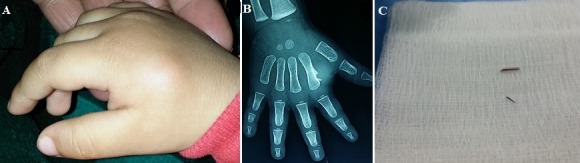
(A) Aspect clinique de la tuméfaction; (B) radiographie de la main montrant la réaction périostée; (C) épines végétales juxta-osseuses extraites
